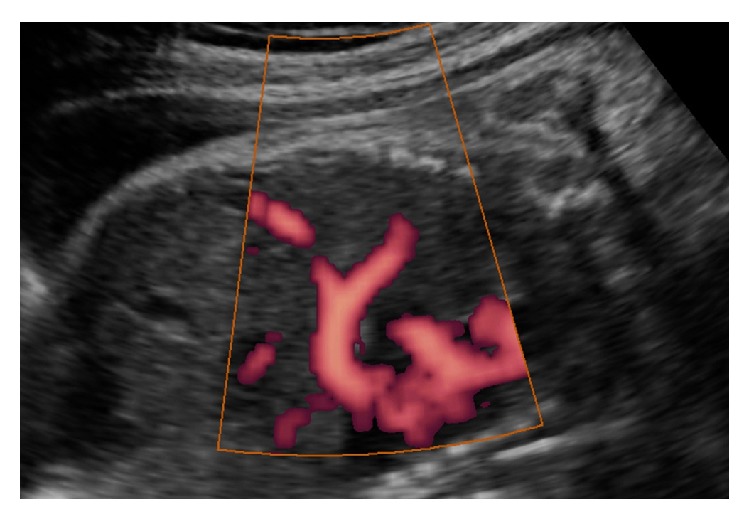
Two-dimensional ultrasound finding in a fetus with ring chromosome 15 at 19 weeks of pregnancy showing liver herniation into the thorax due to congenital diaphragmatic hernia.
